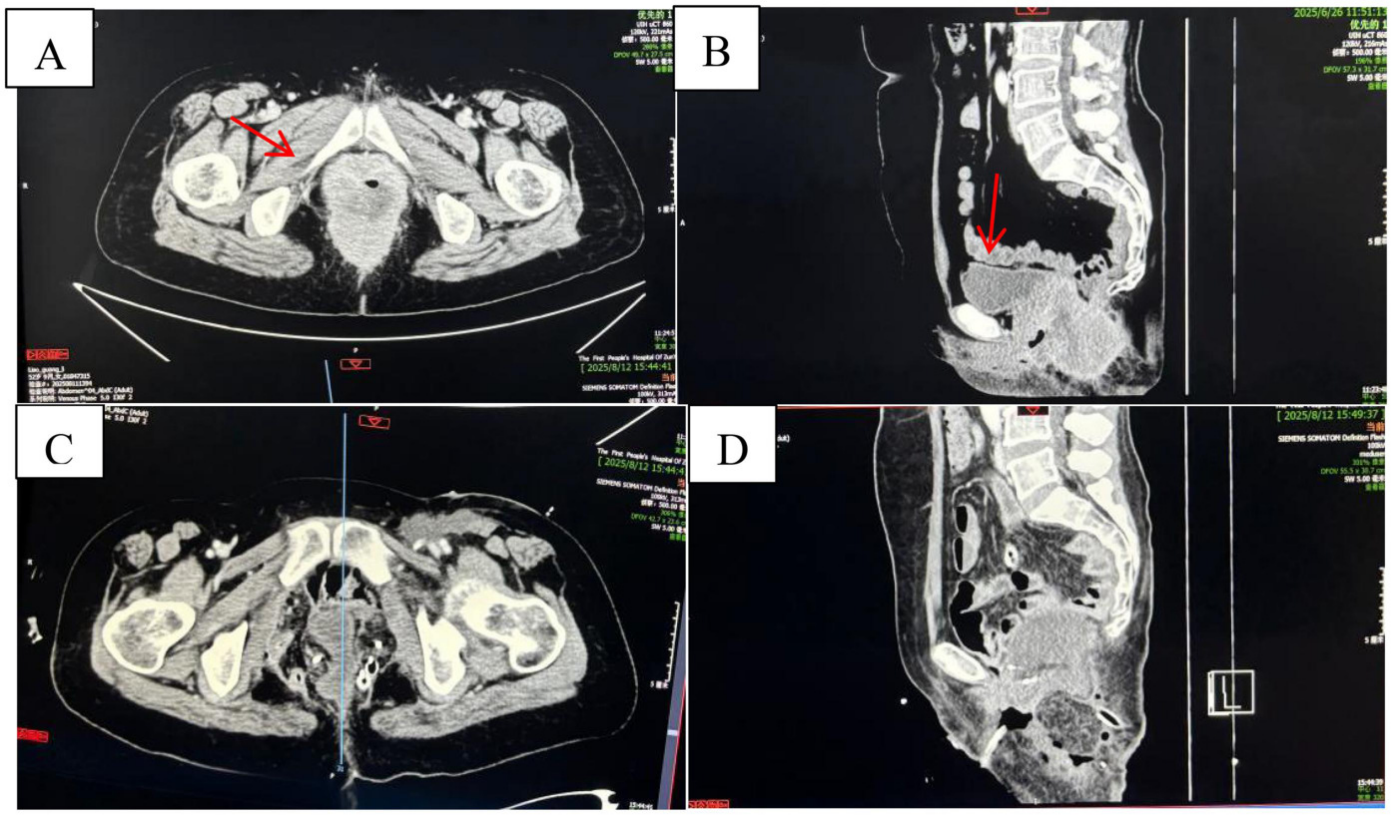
Pelvic CT: (A) preoperative (red arrow): An unevenly thickened soft tissue mass shadow was seen around the rectum-anal canal, with a maximum diameter of approximately 5.5 × 5.3 cm, unclear boundaries, uneven enhancement after contrast administration, and an unclear boundary with the adjacent vaginal wall. (B) Preoperative (red arrow): The bladder wall was smooth with uniform thickness, and no abnormal density lesions were found in the bladder cavity. (C,D) Postoperative: The irregular mass shadow around the rectum-anal canal was resected, and no other obvious abnormalities were observed.
